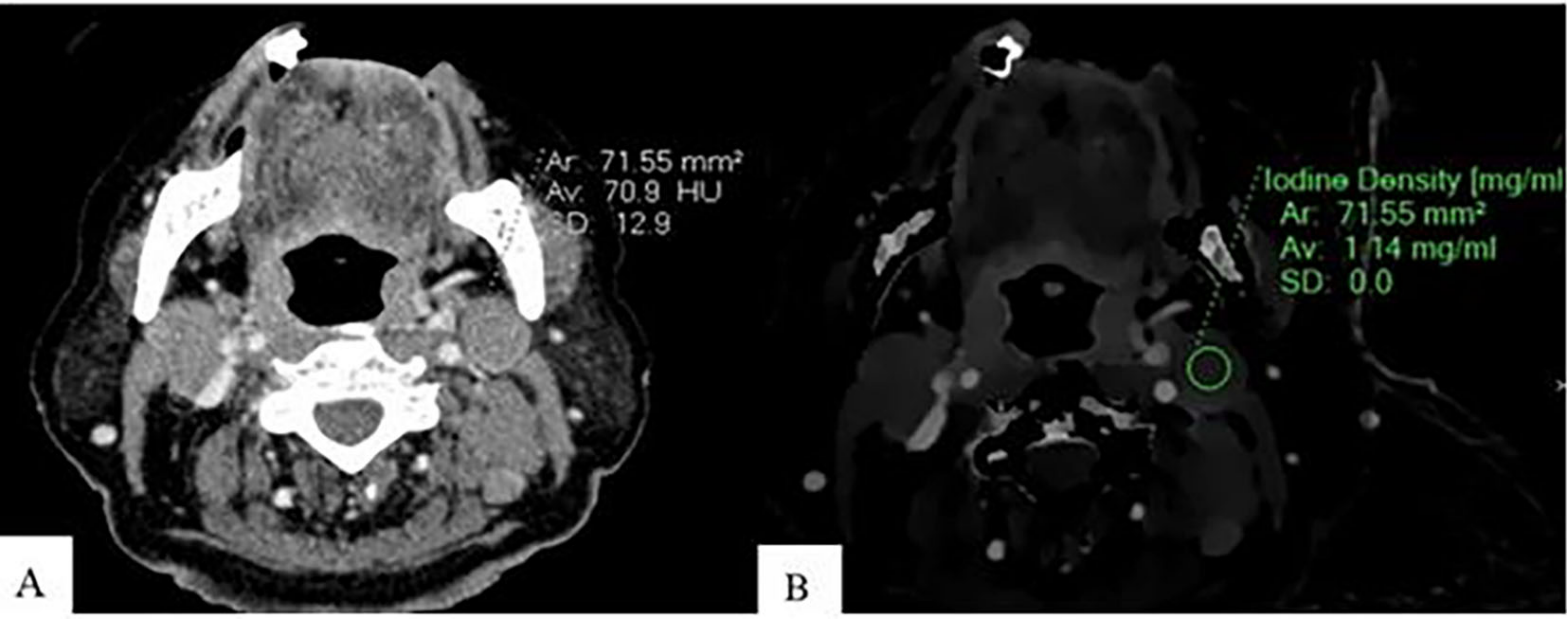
Measurement of ID and CEAV in lymphomatous node. An Axial CT image of a patient with lymphoma shows CEAV measurement (70.9 HU) of the enlarged lymph node at left cervical level IIA (A). An ID measurement (1.14 mg/ml) of this lymph node at the same CT level (B).
